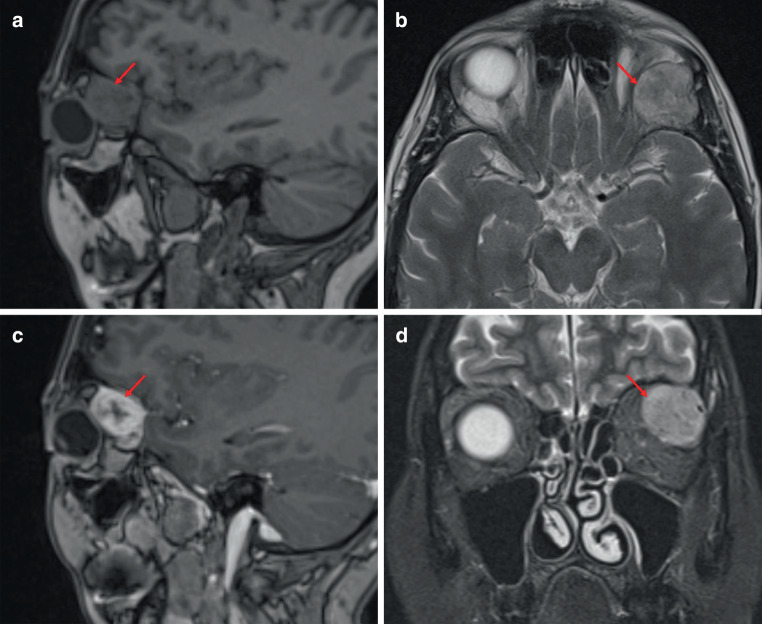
Magnetic resonance imaging of solitary fibrous tumor (SFT) in the laterocranial quadrant and extraconal space of the left orbit in a 57-year-old woman. a sagittal plane of non-contrast T1-weighted imaging with SFT (*red arrows in all images*), b axial plane of T2-weighted imaging, c sagittal plane of contrast-enhanced T1-weighted imaging, d coronal plane of STIR imaging. *SFT* solitary fibrous tumor, *STIR* short time inversion recovery
